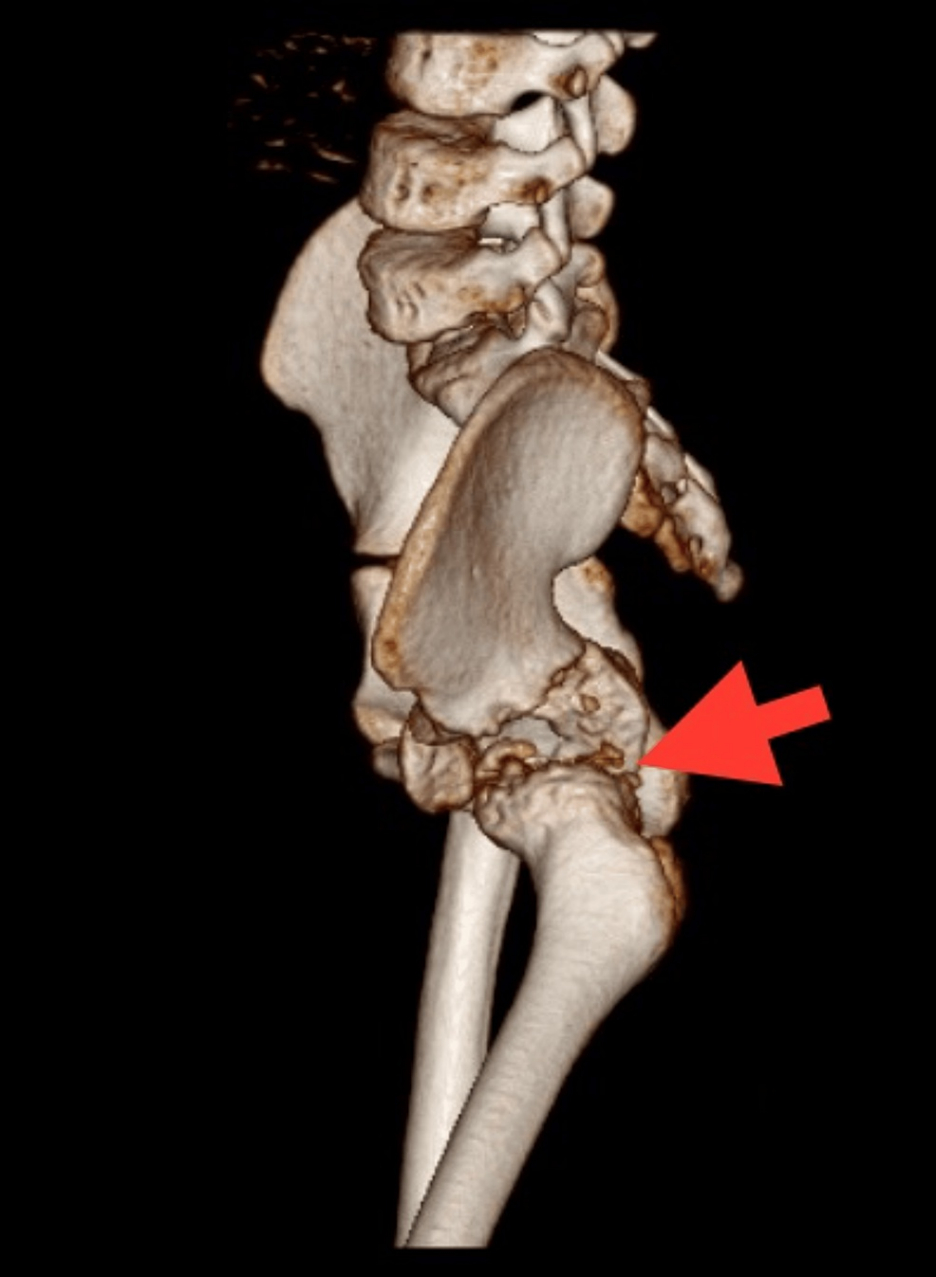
3D CT scan of the lateral view of the left hip joint 3D: three dimensional; CT: computed tomography
